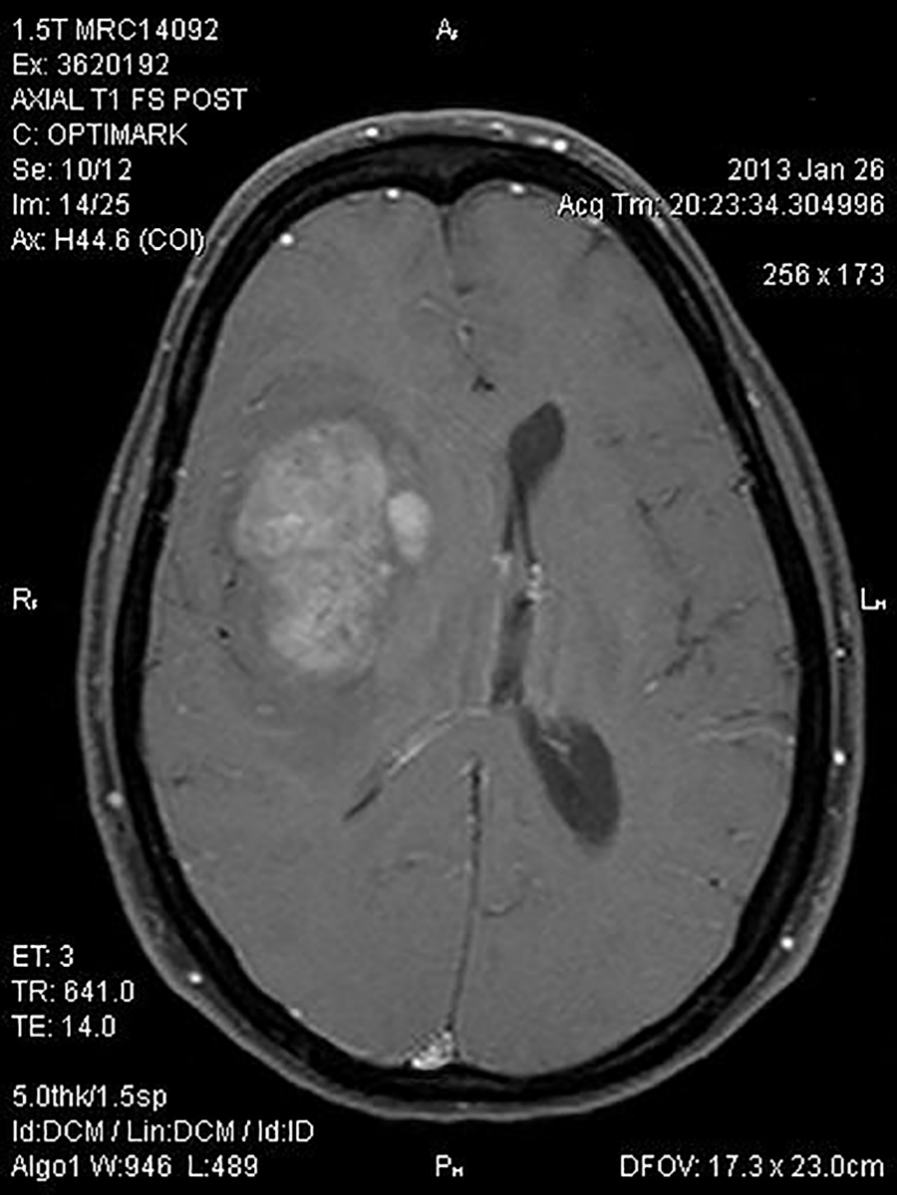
Initial MRI of the brain with and without contrast. A large right frontal lobe intra-axial mass with mass effect upon the right ventricular system and a leftward midline shift of approximately 1.3 cm is shown.
